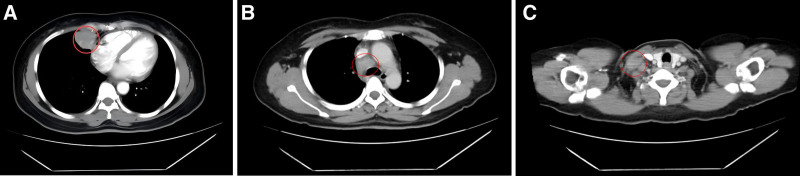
Baseline chest CT. (A) Lesion in the right lung; (B) enlarged mediastinal lymph nodes; (C) metastatic lymph node in the right supraclavicular region. CT = computed tomography.
